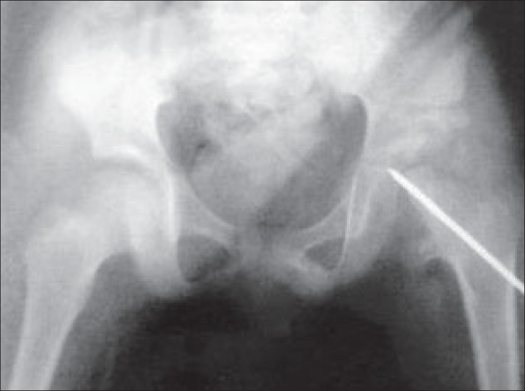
X-ray eight weeks after surgery shows reduced proximal femur with a pin
